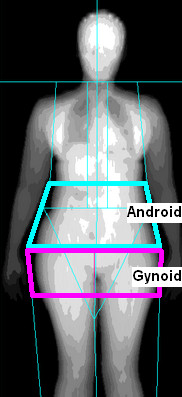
Dual-energy X-ray absorptiometry whole-body scan. Android region outlined in blue and gynoid region outlined in pink.
